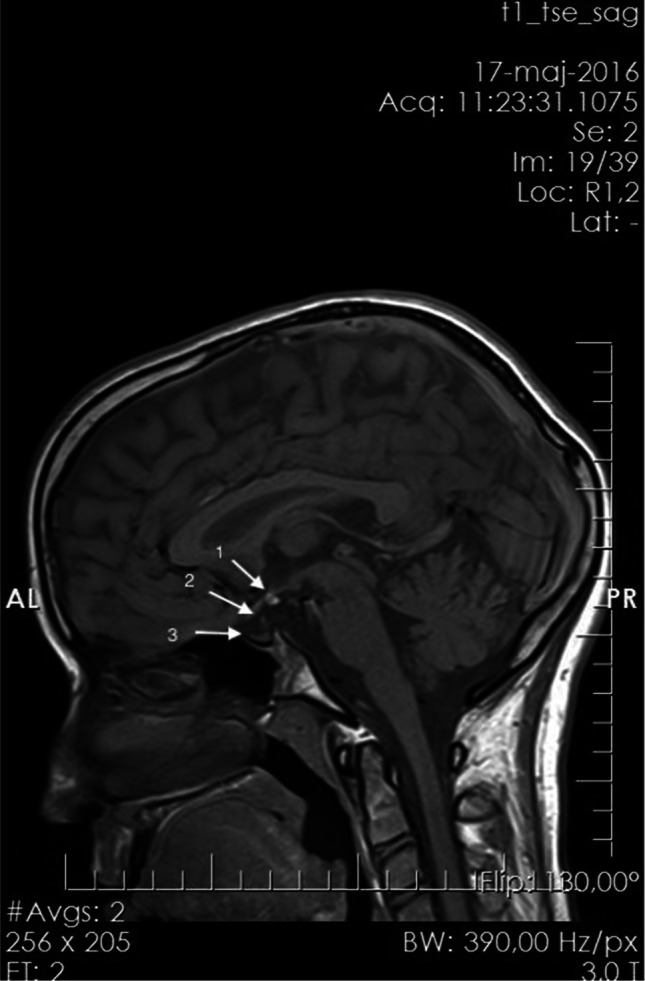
MRI image (sagittal plane) of the head of the presented patient showing typical triad of PSIS: ectopic posterior lobe (1), agenesis of the pituitary stalk (2), and hypoplastic anterior lobe (3). This examination came when the girl was 17 years old
